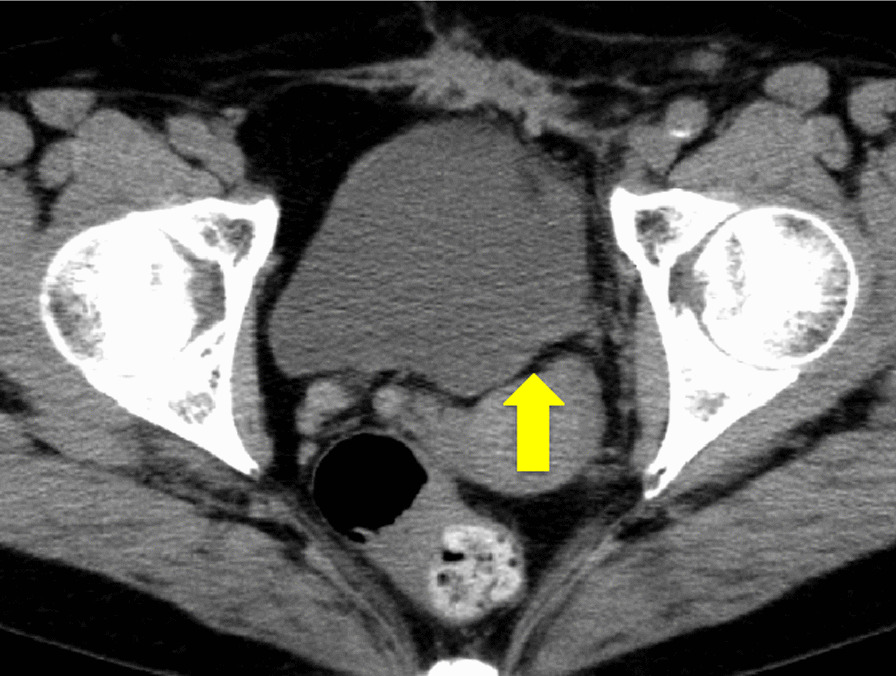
Postoperative computed tomography showed no recurrence (tumor: arrow)
